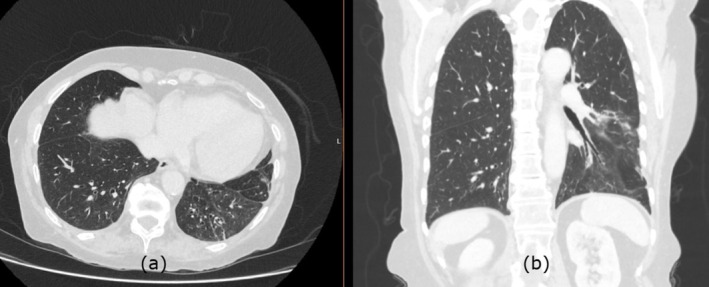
The CT scan following the treatment with steroids showing near complete resolution of the left lower lobe consolidation with minimal residual reticulation both in axial (a) and coronal images (b).
